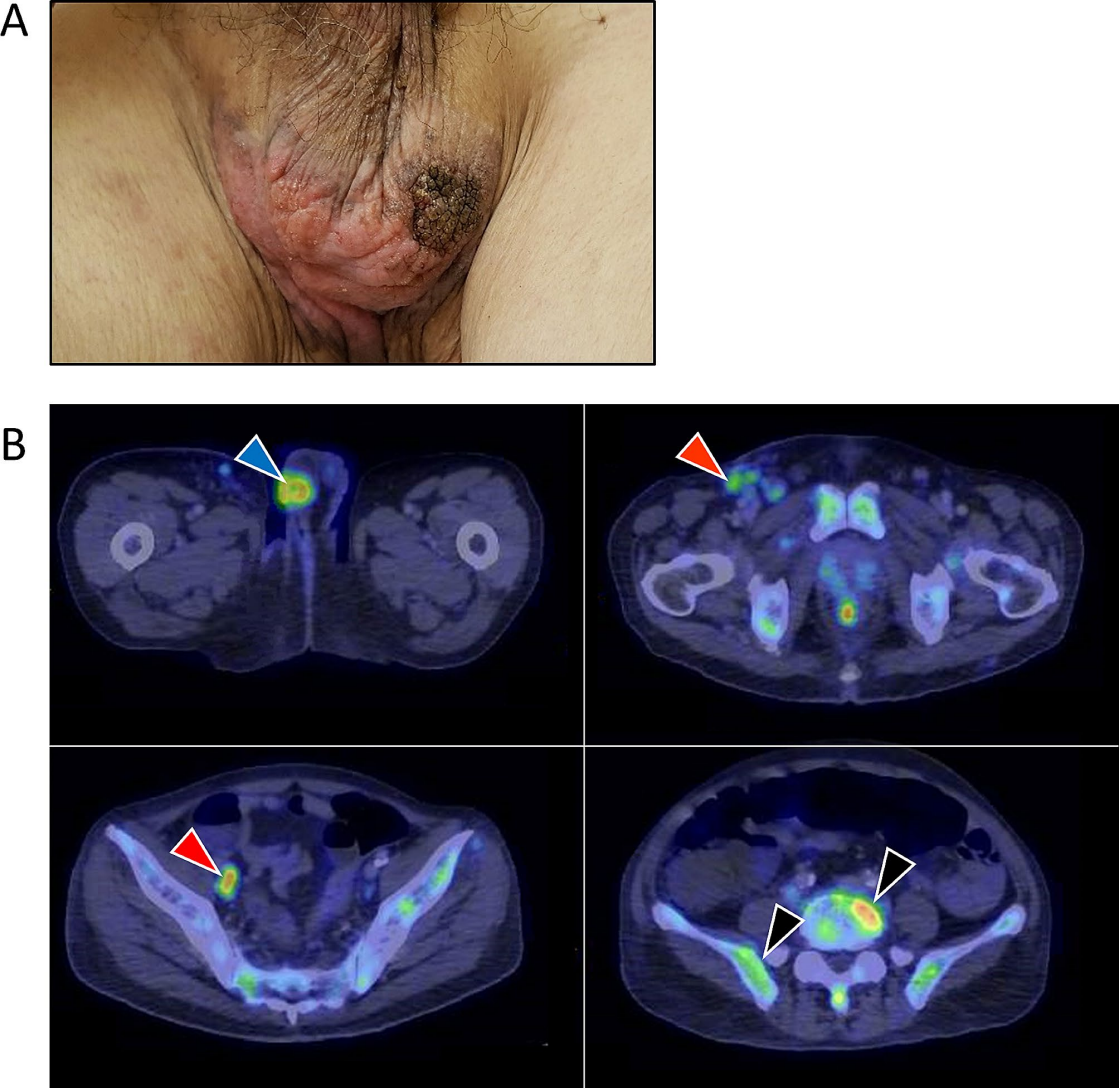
Clinical and diagnostic features of the patient with metastatic EMPD. (A) Erythematous hyperkeratotic rash with central ulceration over the scrotum. (B) PET/CT imaging. Blue arrow indicates an FDG-avid scrotal wall mass measuring 2.8 cm x 2.5 cm (SUVmax 6.9). Red arrows indicate right iliac and inguinal lymph nodes involvement (SUVmax 6.7). Black arrows indicate extensive FDG-avid mixed lytic-sclerotic bony lesions involving the ribs (not shown), spine and pelvis (SUVmax 9.3)
